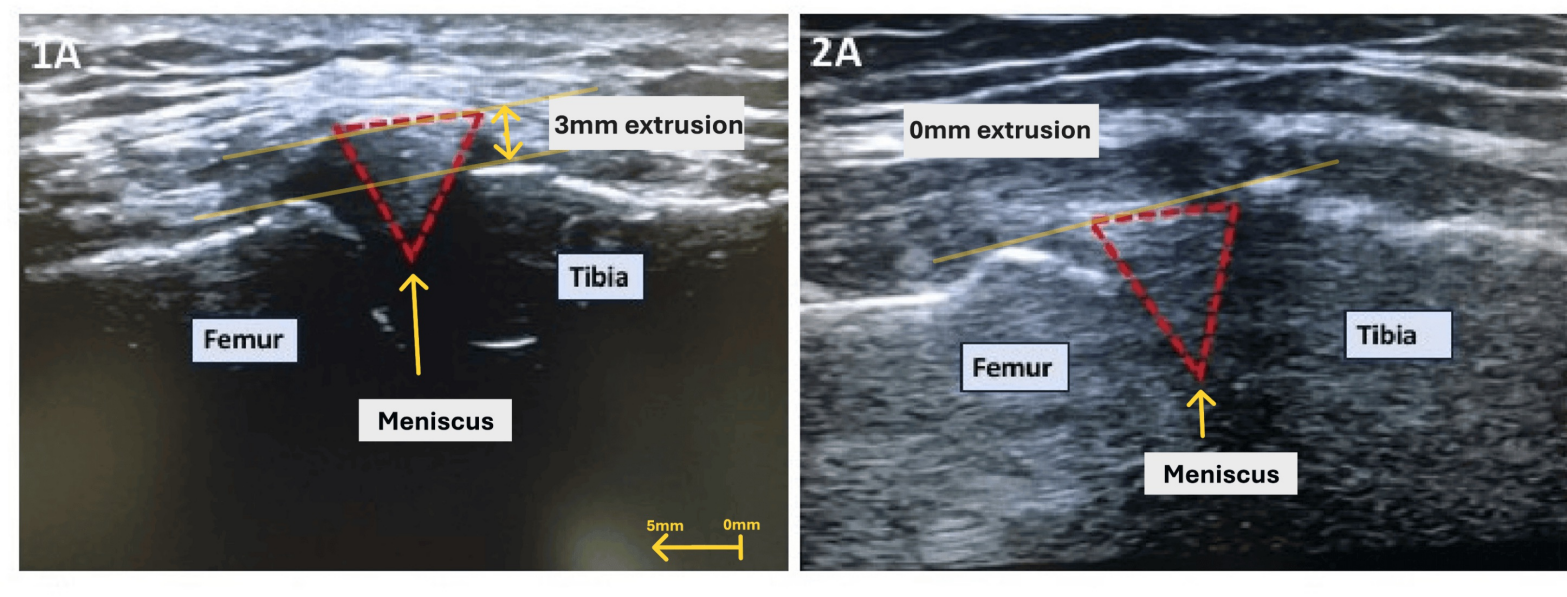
Static ultrasound image of a medial meniscus showing an extruded meniscus by 3 mm when the compartment is loaded (A) and an image taken two weeks postoperatively following root repair showing a reduced and stable meniscus (B). Image Source: Tarek Boutefnouchet
